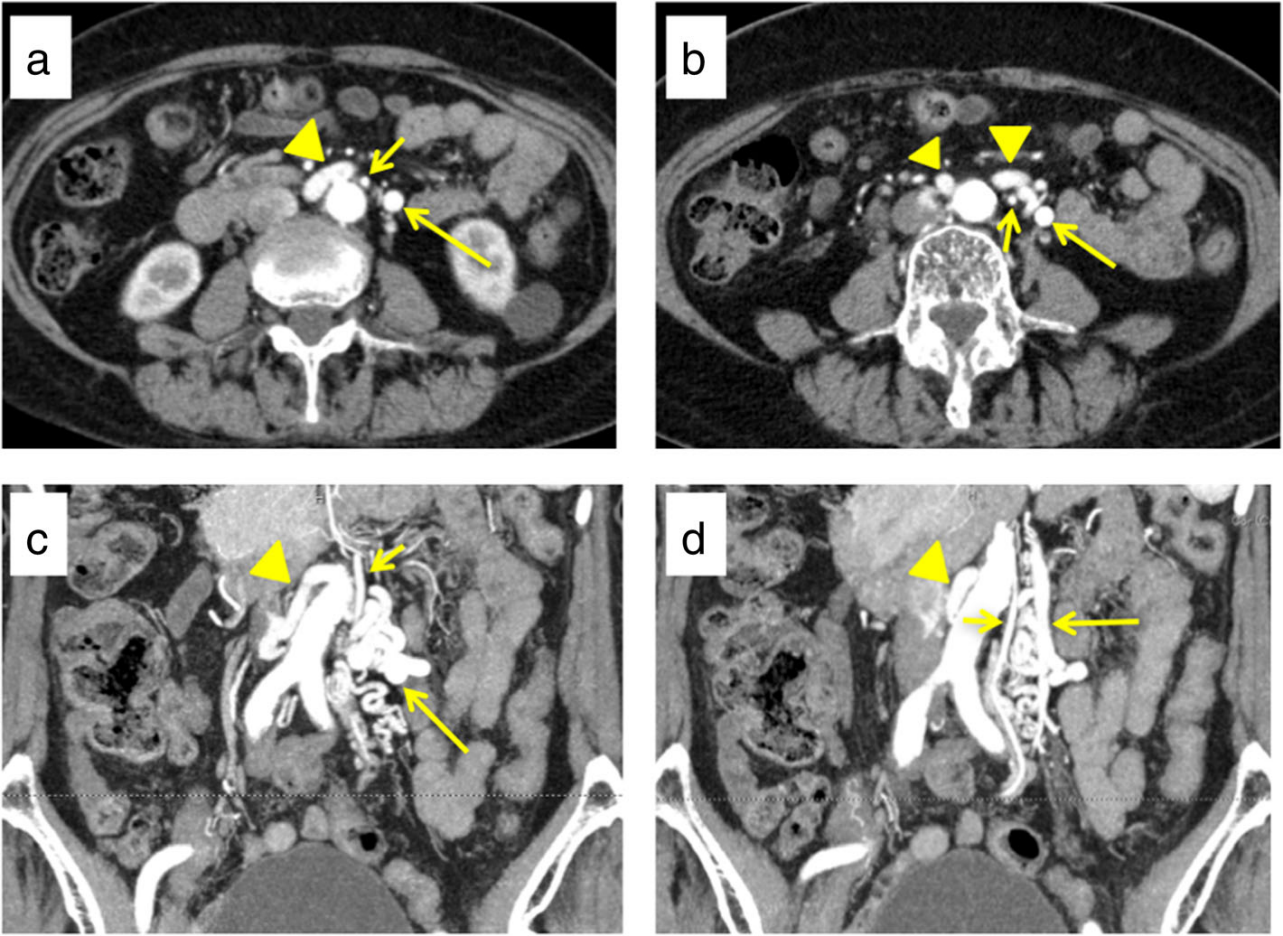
An abdominal contrast CT scan shows a venous malformation forming a short circuit (Retzius shunt) from the IMV to the IVC. a, b Axial views. c, d Coronal views. Retzius shunt (arrow head). IMV (long arrow). IMA (short arrow)
